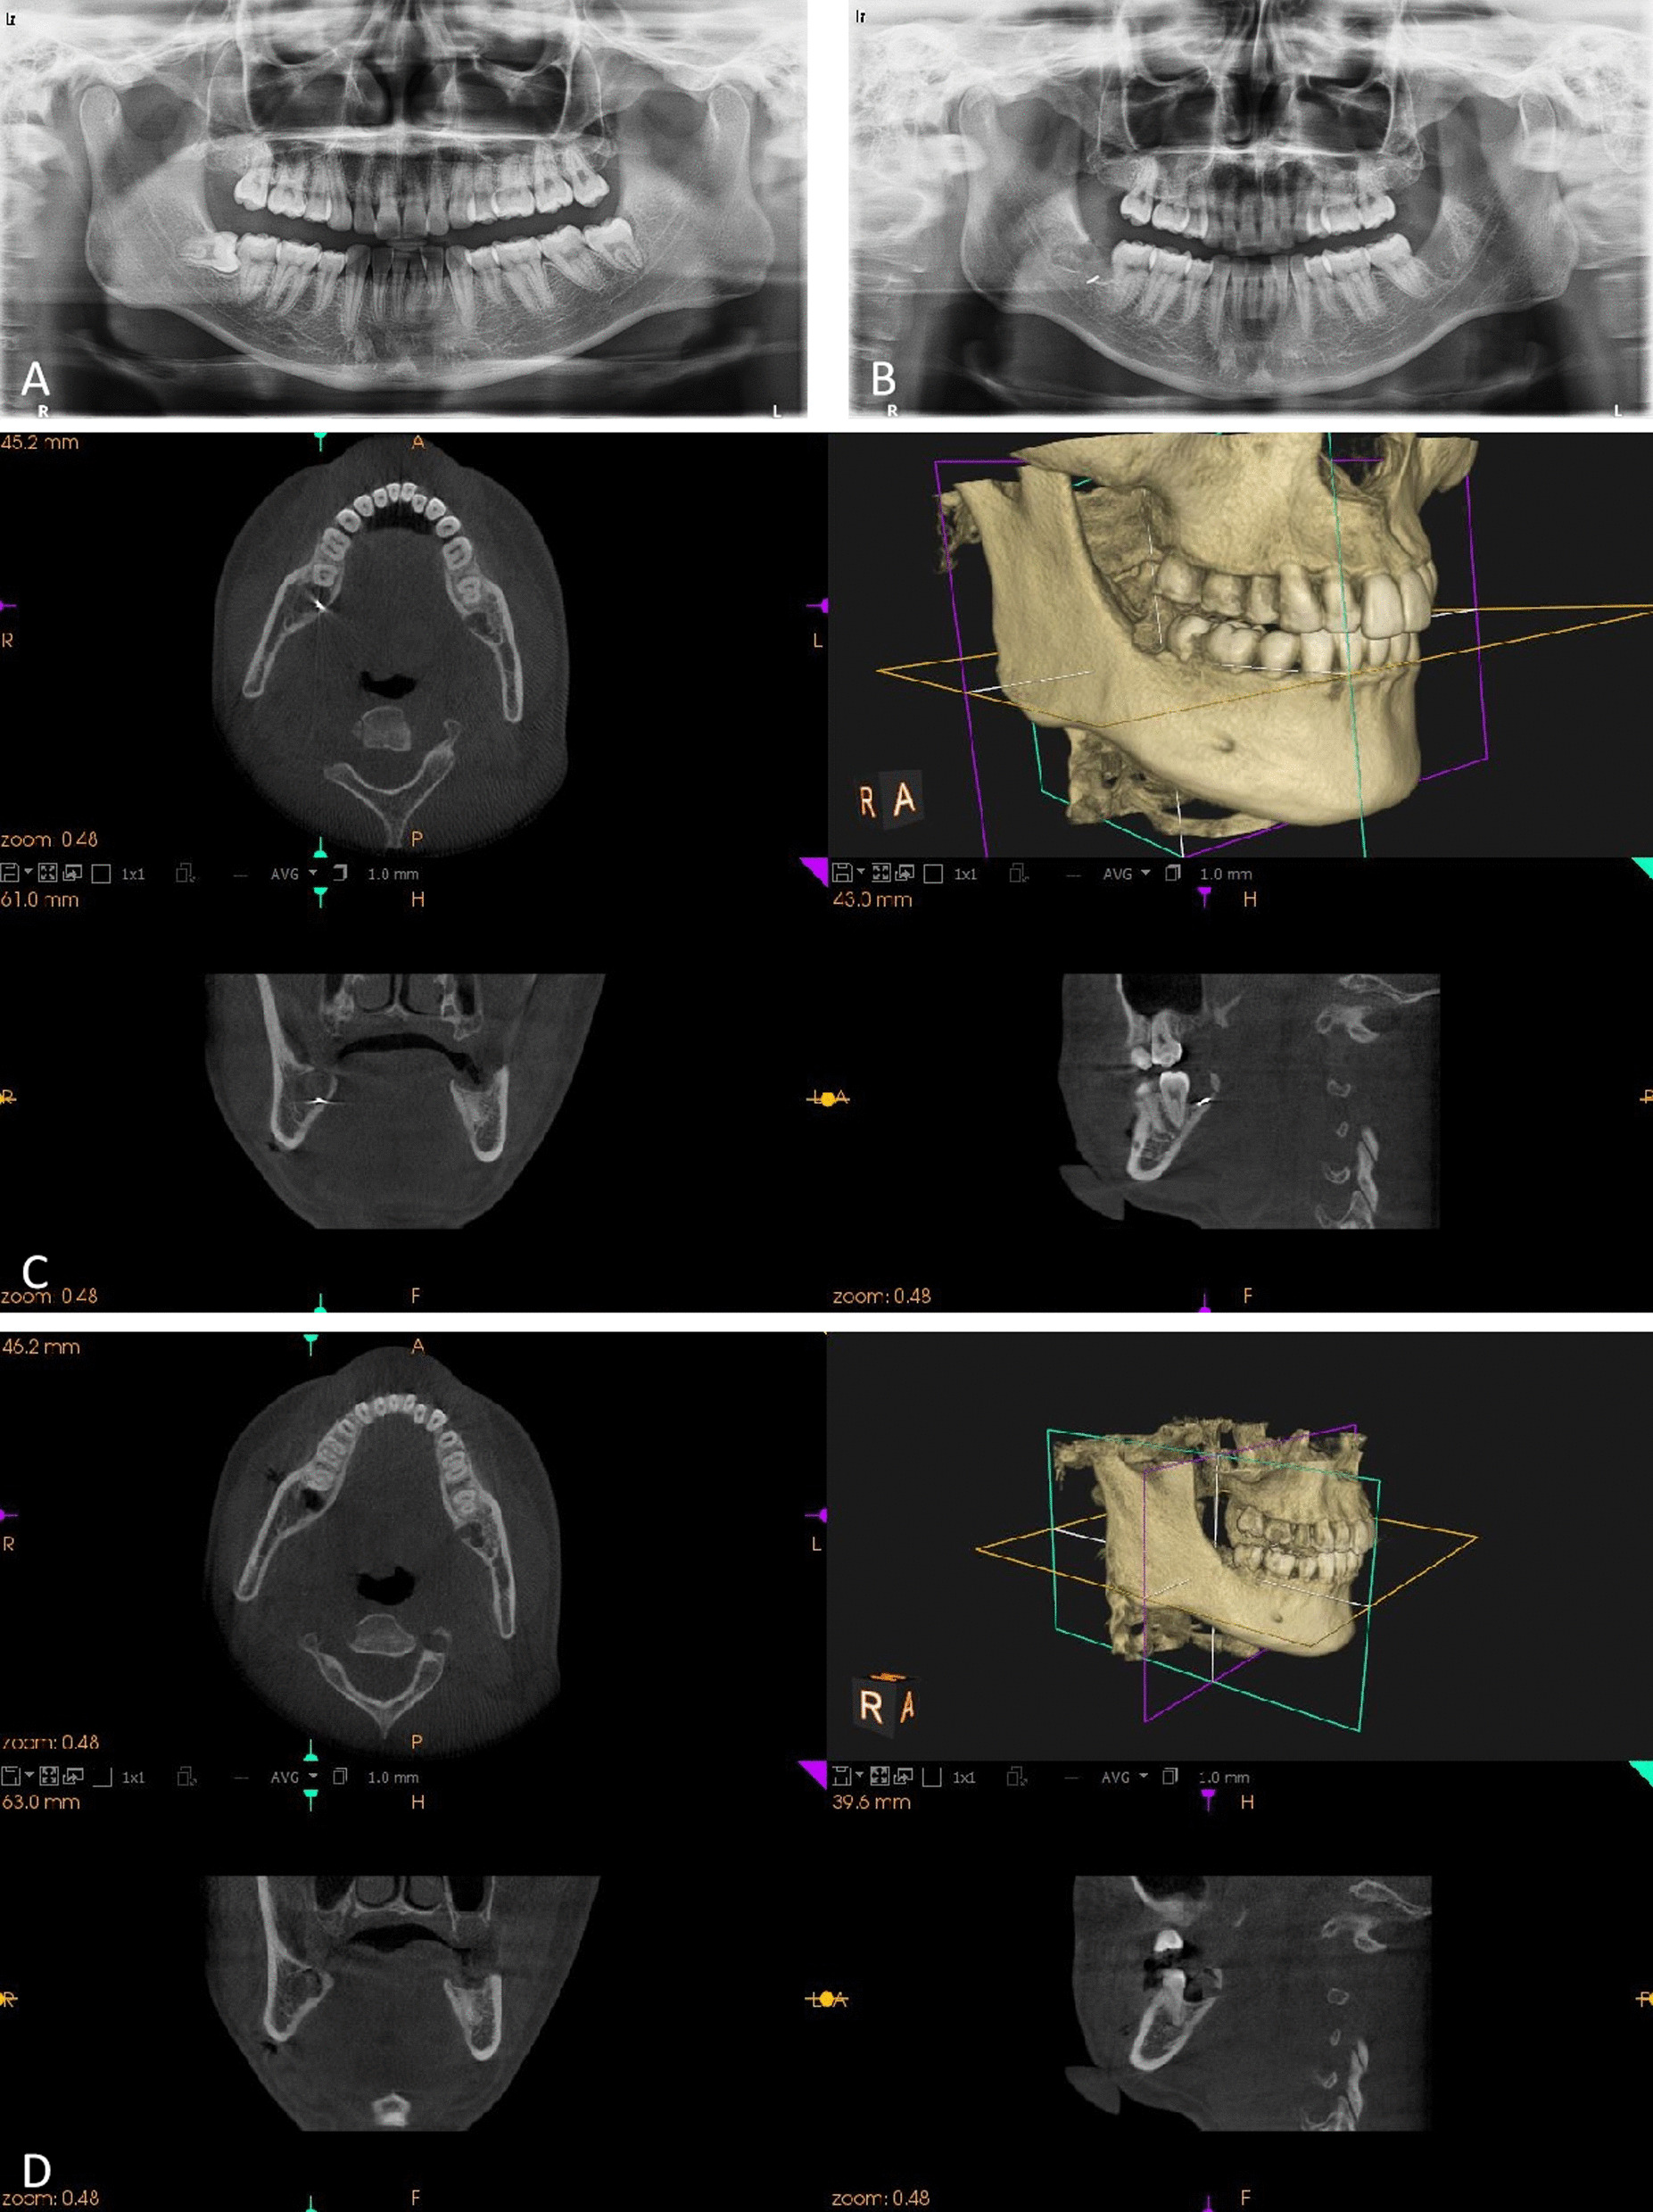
Case 1* A Preoperative panoramic radiograph. B Panoramic radiograph showing the bur fragment in the socket of the right mandibular third molar. C CBCT showing the 3D position of the bur fragment. D CBCT confirmed the removal of the bur fragment
